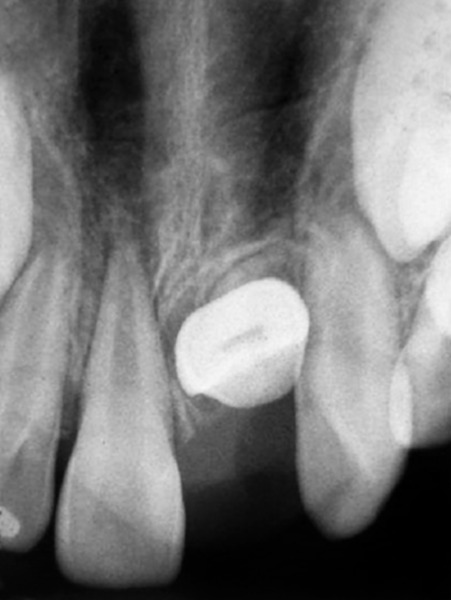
Preoperative IOPA radiograph
